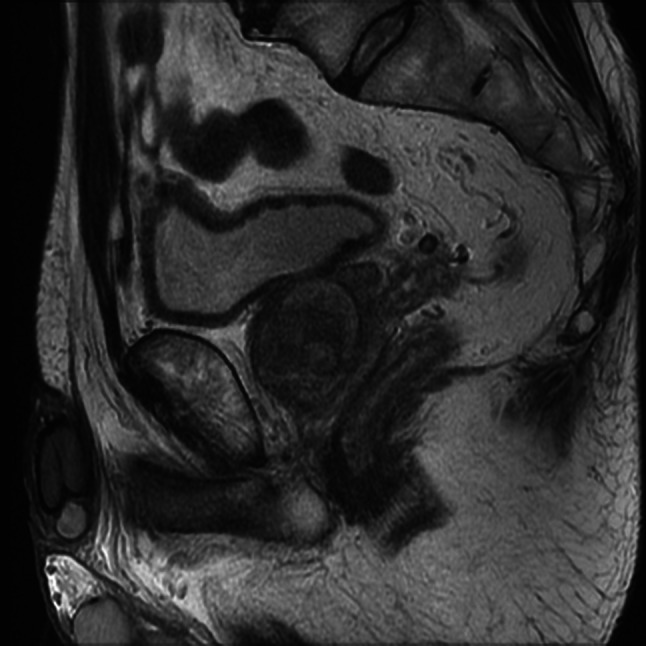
Plain MRI image of the bladder after PCG therapy. The tumor has shrunk and the bladder wall thickening has decreased
